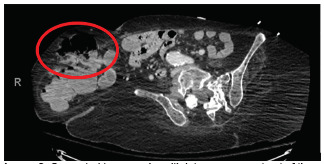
Computed tomography with intravenous contrast of the abdomen and pelvis. No active extravasation was found, and in the circled area above in the parastomal hernia (large red circle).
